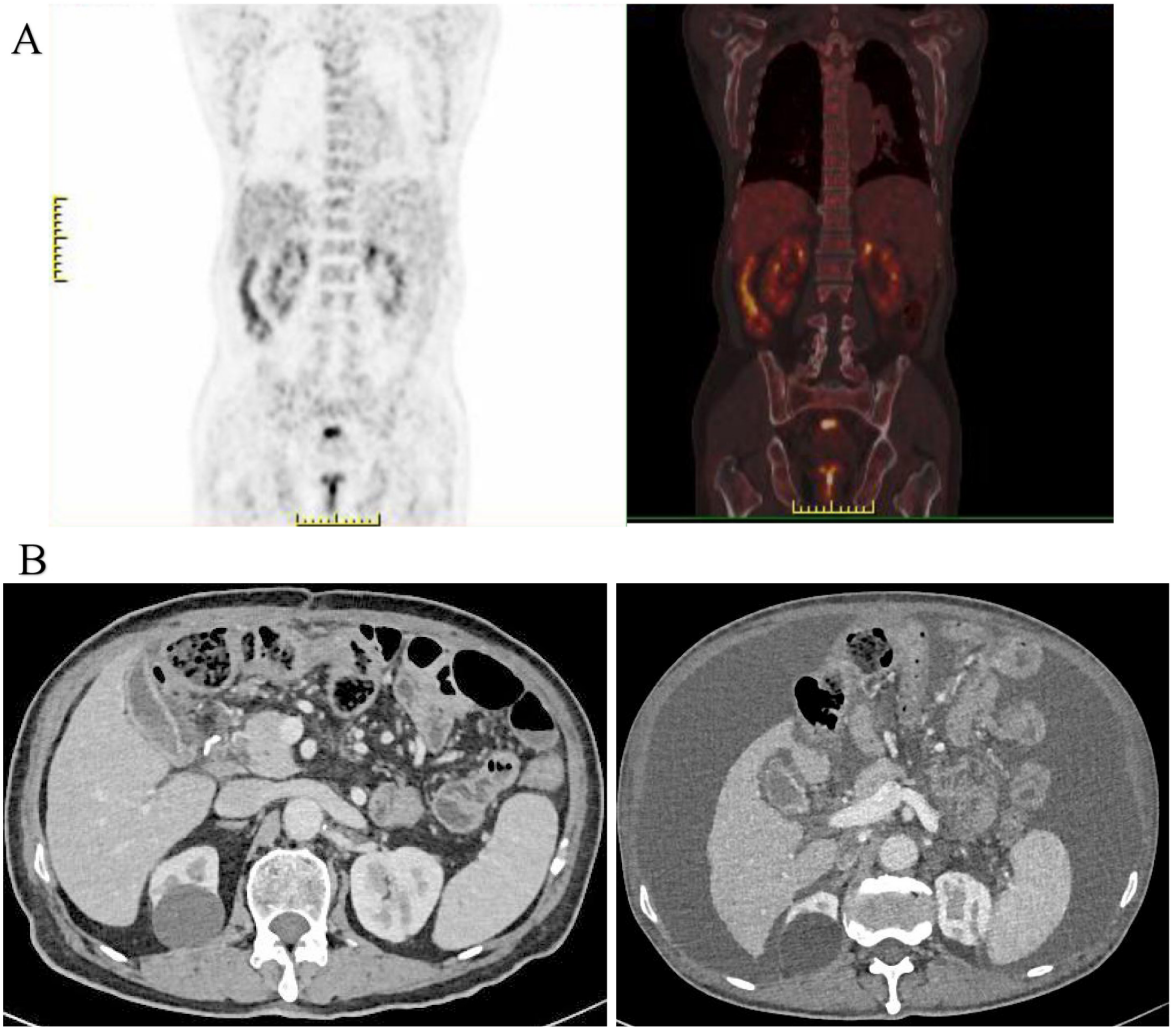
(A) PET-CT imaging demonstrated no findings suggestive of tumor recurrence; (B) Enhanced abdominal CT revealed a significant accumulation of fluid in the abdominal cavity compared to previous findings (August 2023).
